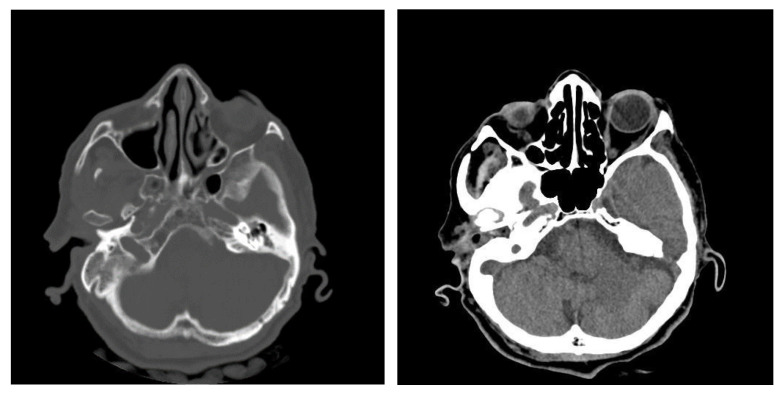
Patient with diagnosis of right suppurative chronic polypous polypous otomastoiditis. CT examination in axial sections shows absence of mastoid cell pneumatization and presence of a tissue mass in the right external auditory canal.
